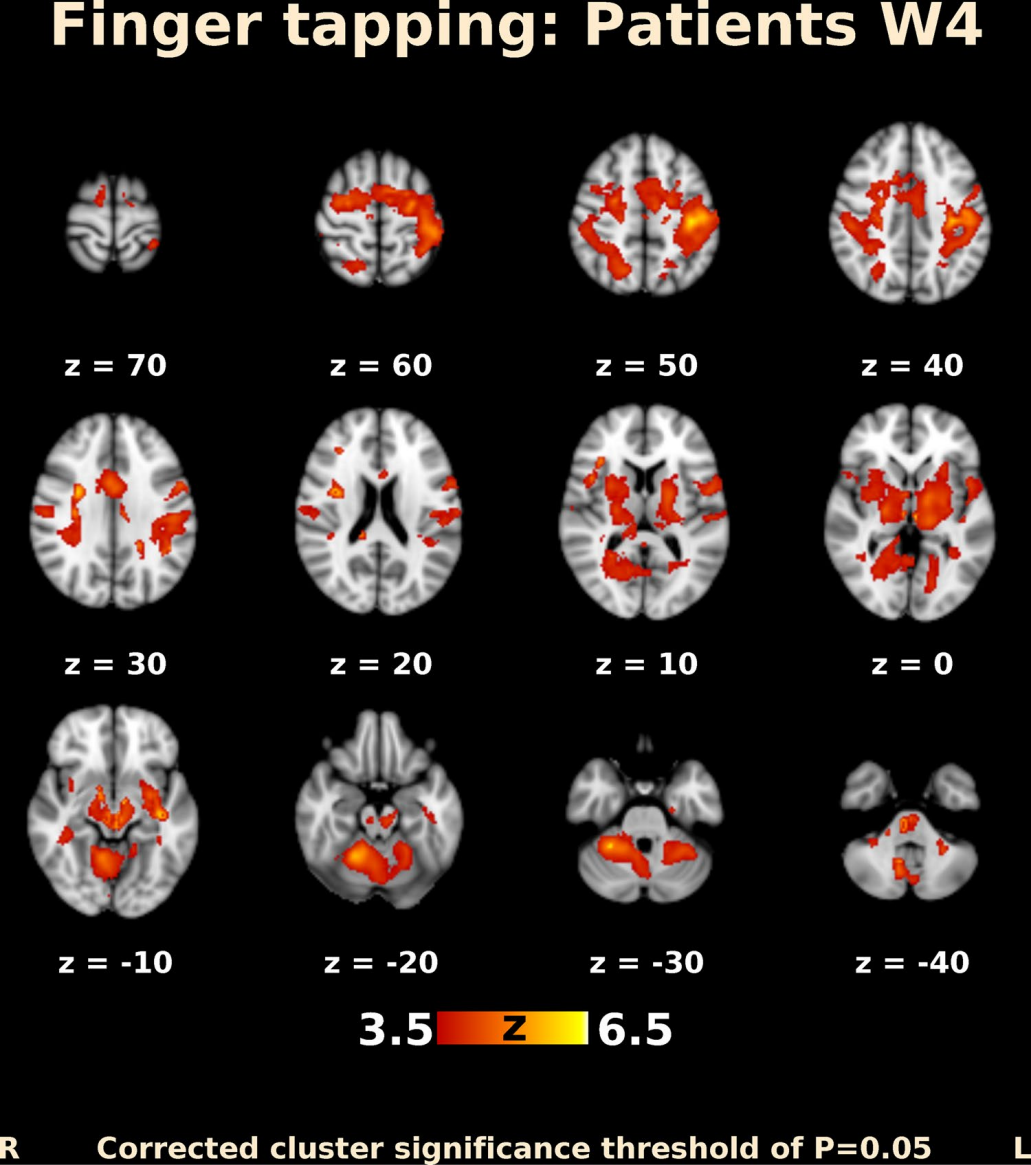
Functional MRI activation map in patients with CD 4 weeks after BoNT-A injection. Slices are labeled with *Z*/*Y* coordinate in standard MNI152 space
